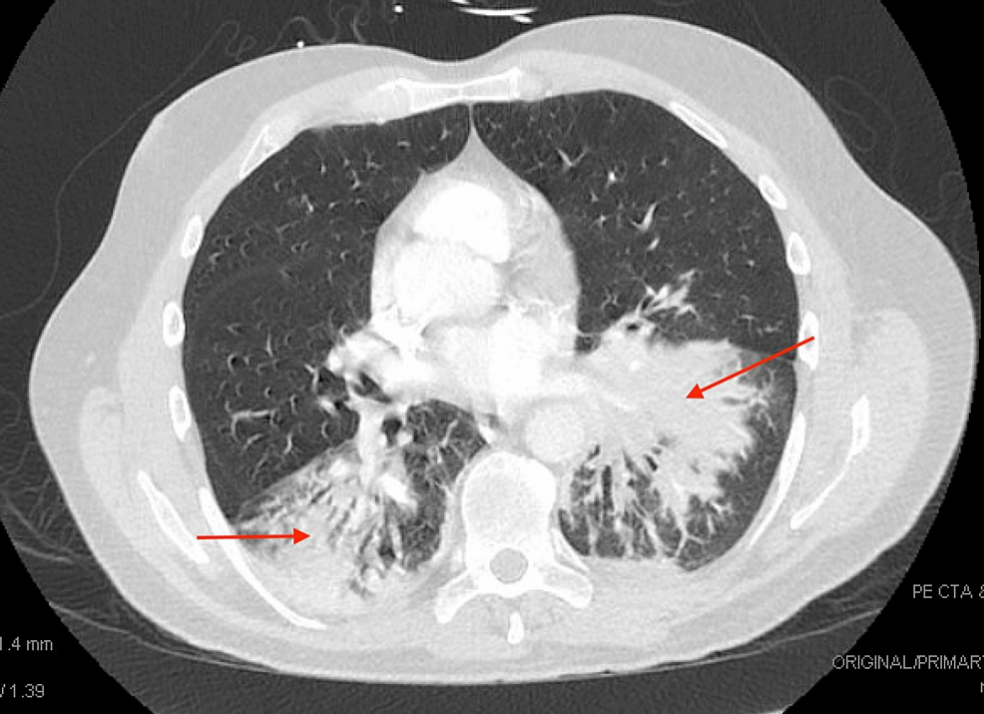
CT chest showing bilateral airspace opacities
